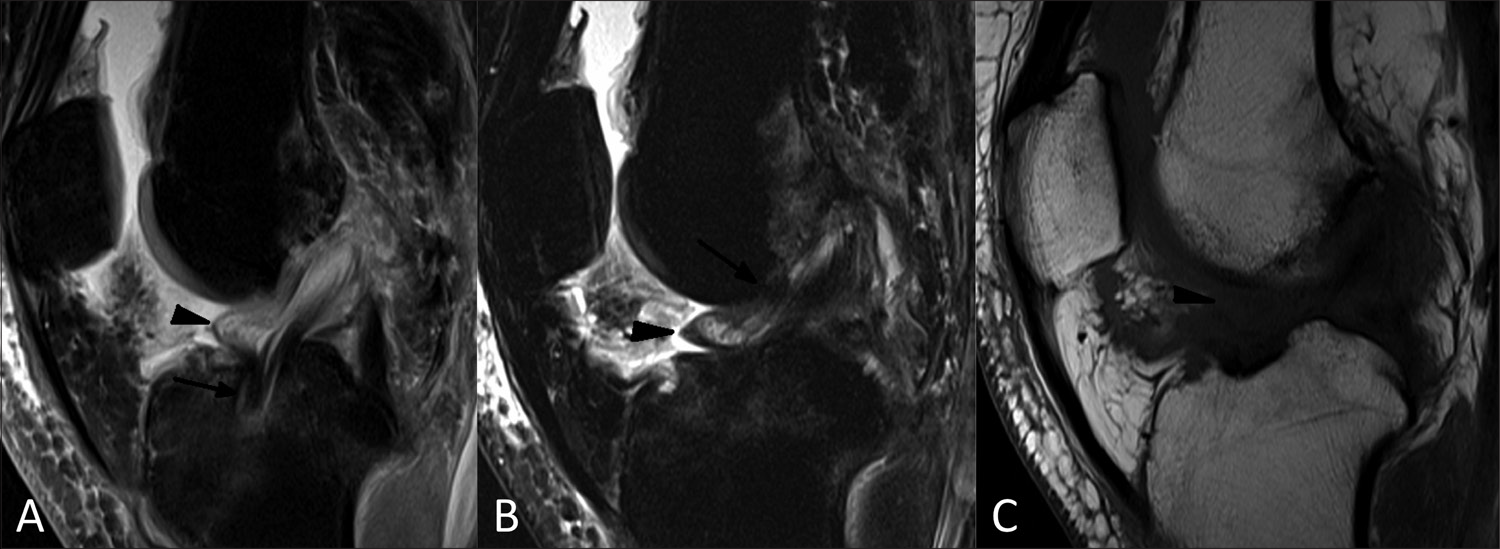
(A-C) Case 2: Fat-suppressed sagittal proton-density weighted (A) and T2W (B) MRI images show a hypointense to isointense nodule (arrowhead) attached to the anterior surface of the anterior cruciate ligament (ACL) graft (arrow). Sagittal T1W MRI image (C) shows a hypointense nodule (arrowhead) in the anterior intercondylar notch
